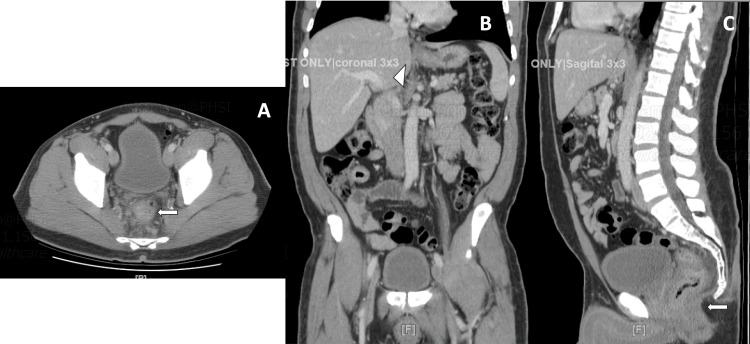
CT of abdomen and pelvis Images showing hepatic steatosis with a normal caliber of the biliary system (arrowhead), focal moderate wall thickening of the rectum (arrows), and multiple enlarged perirectal adenopathy (A. Axial; B. Coronal; C. Sagittal)
